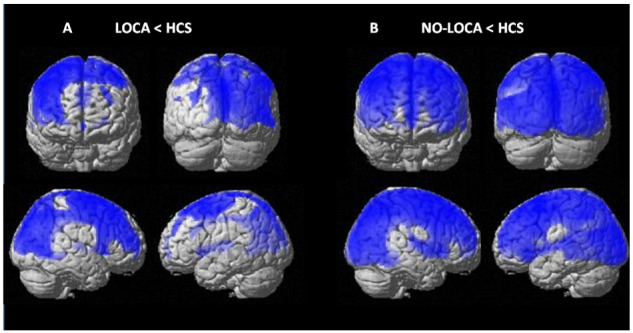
^18^FDG-PET results. Areas showing significant metabolic impairment (in blue) in A NO-LOCA and B LOCA patients in UWS compared with HCS, thresholded at *P* < 0.05 FDR-corrected for multiple comparisons (at the whole-brain level).
